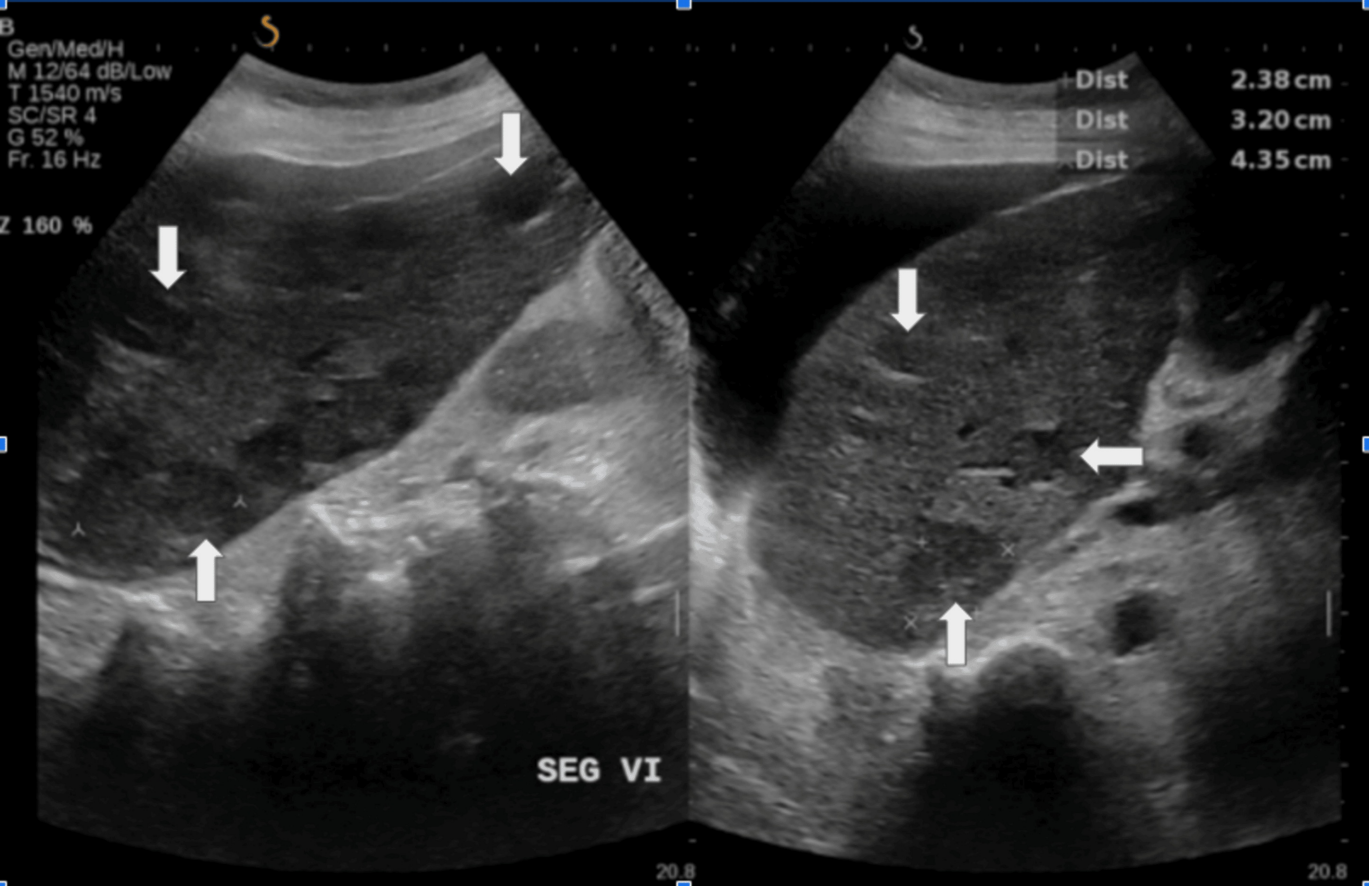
Ultrasound of the hepatobiliary system showing multiple hypoechoic liver lesions (largest 4.0 × 3.2 cm) in the background of liver cirrhosis.
